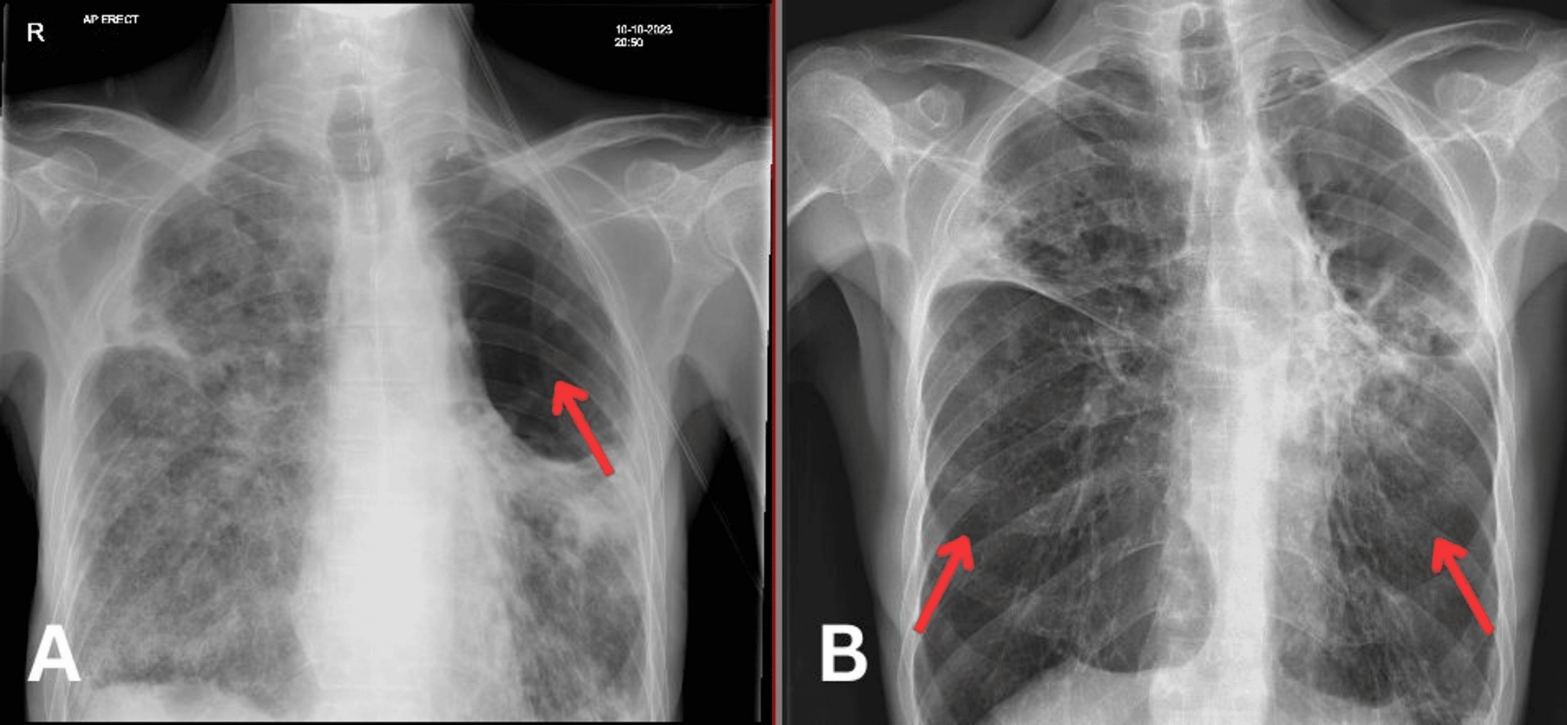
Chest X-ray during the first admission (A) and second admission (B).
